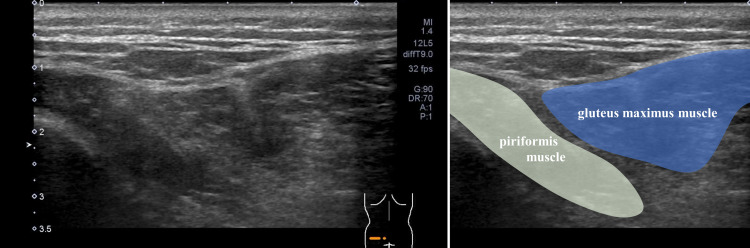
Muscle ultrasonography. Short-axis image of the gluteal and piriformis muscles.
